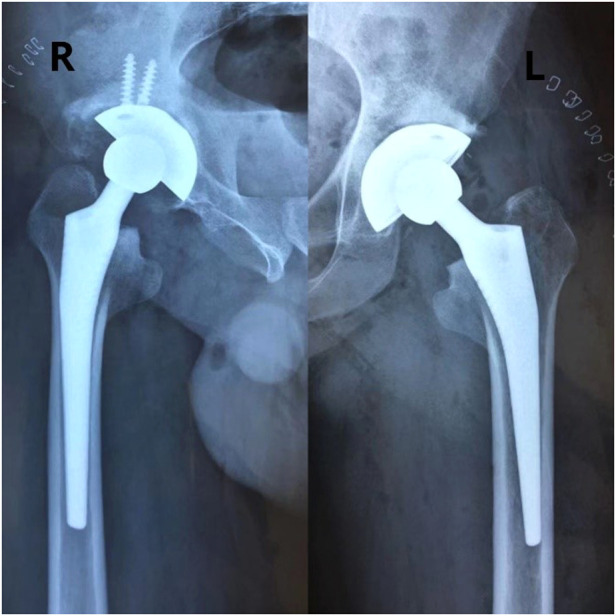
Patient's hip x-ray 1 month after surgery. (Figure notes: X-rays showed that the bilateral hip prostheses were well positioned, with no obvious dislocation or other adverse effects).
